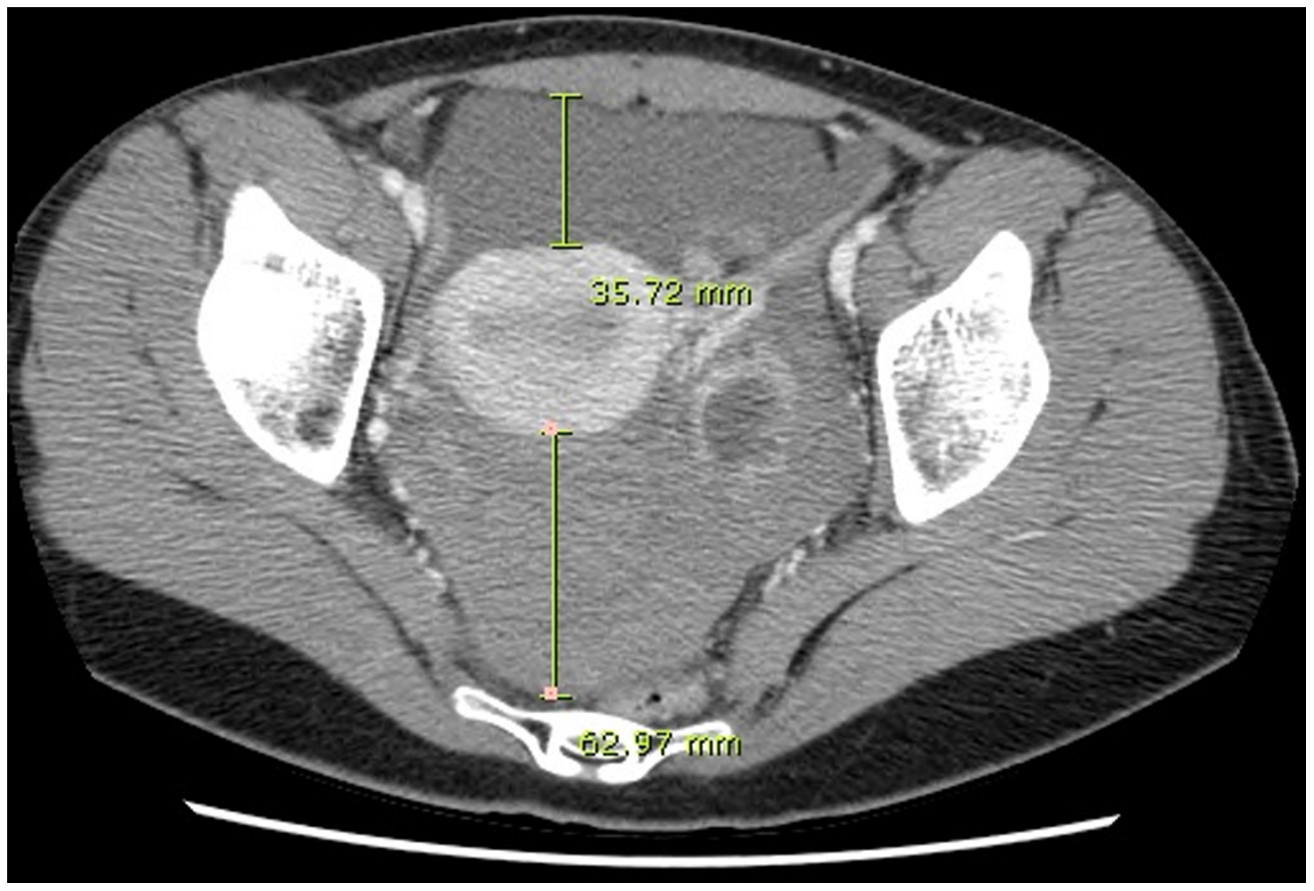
CT quantification of hemoperitoneum. The depth in the anterior cul-de-sac and the posterior cul-de-sac are defined the distance from the anterior peritoneum to the anterior uterine wall and the distance from the posterior uterine wall to the rectum, respectively.
